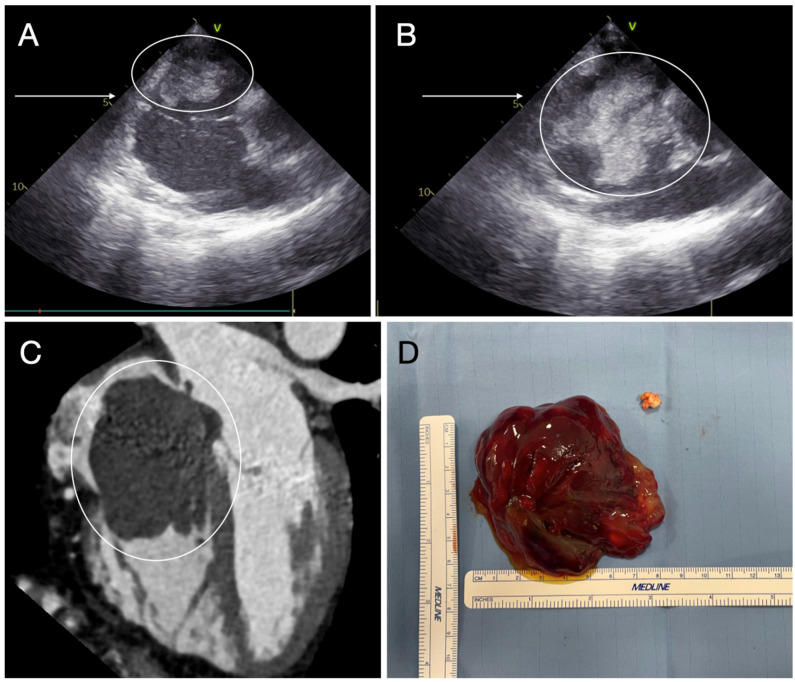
Transesophageal echocardiography shows a polypoid right-atrium mass (encircled) (A,B) adherent to interatrial septum by its pedicle (A), crossing the tricuspid valve (arrow) (A,B) in the diastole (B). Computed tomography reconstruction (C) confirms the characteristics of the mass (encircled) that shows the gross features of a cardiac myxoma after excision (D).
